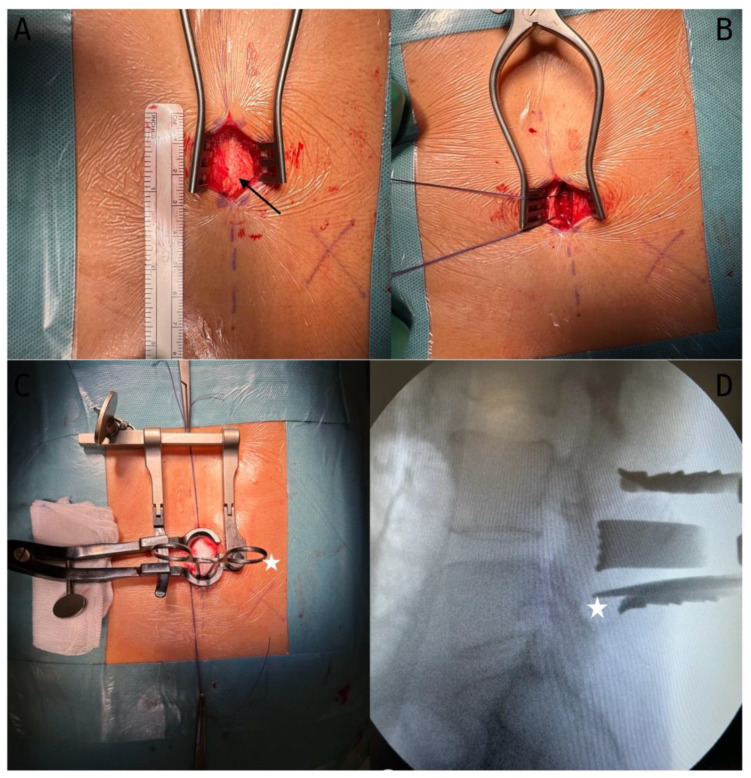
(A) Incision and muscular fascia (black arrow) exposition; (B) muscle fascia handling; (C) skeletonisation of the homolateral lamina and placement of a Caspar distractor. Klemmer forceps are positioned under the lamina for X-ray control (white star); (D) X-ray control of the correct Caspar positioning on the right level.
